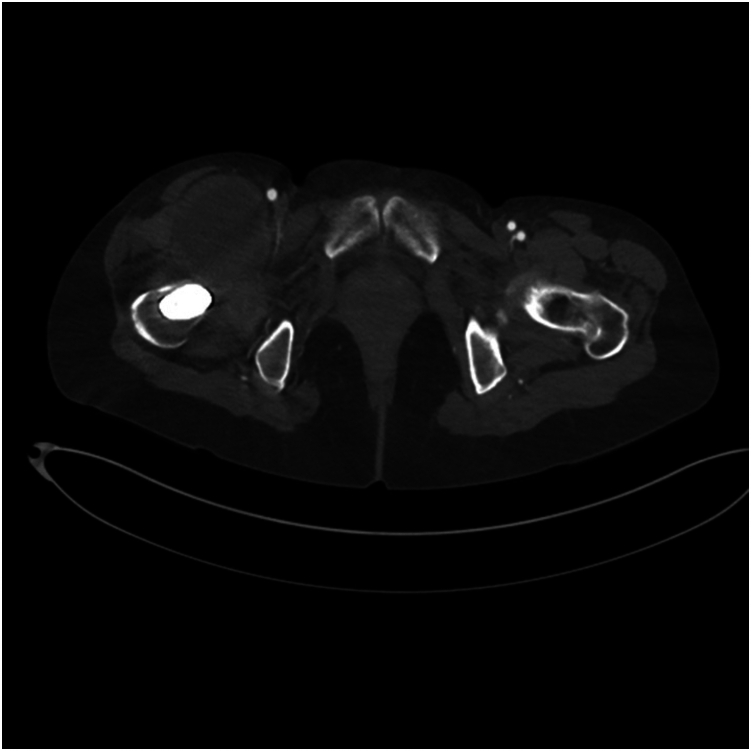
Axial view of right-sided iliopsoas hematoma. CT scan demonstrating a hypodense mass measuring up to 5.4 × 4.9 cm in cross-section and 9.7 cm in craniocaudal dimension.
